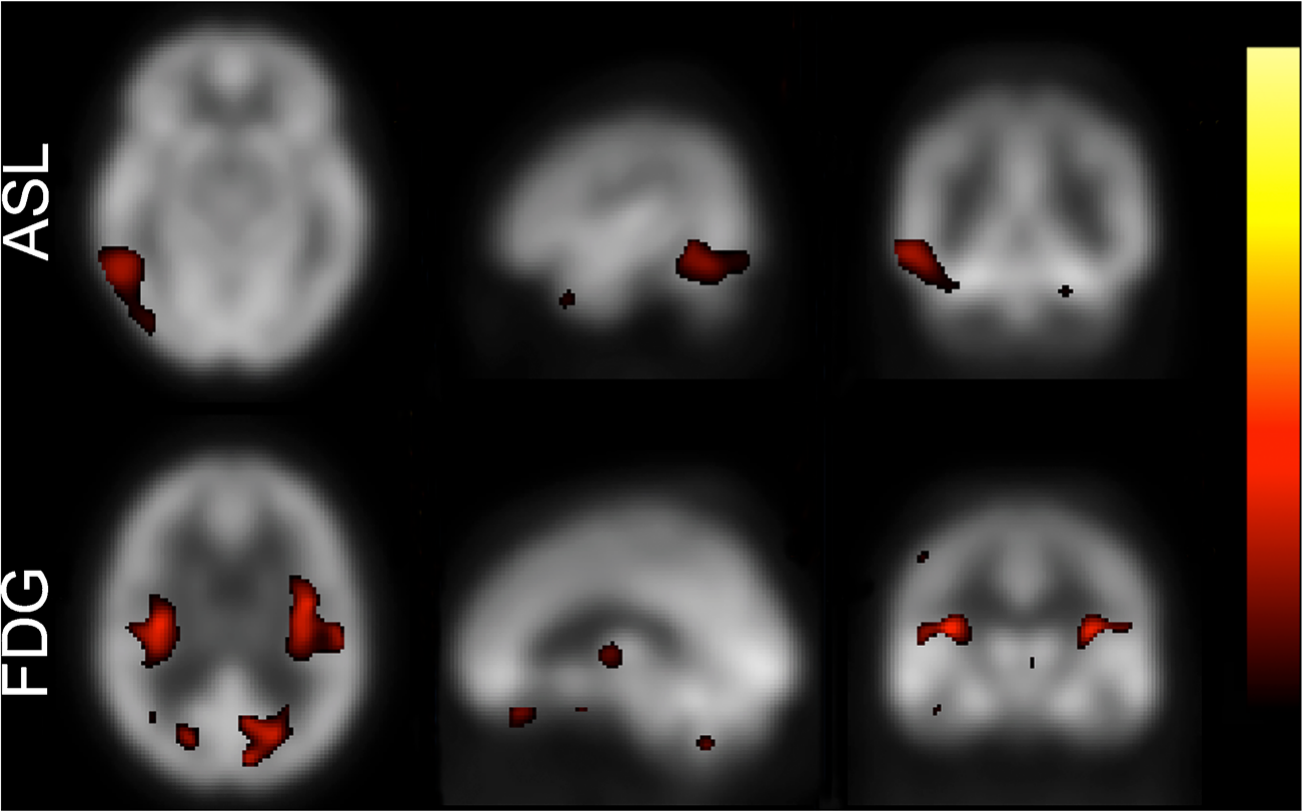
Healthy control with false-positive findings. Both the ASL-based and FDG-PET-based Z-score maps show false-positive Z-score patterns representative for the respective modality in this study. The clusters at the junction between grey and white matter in the lower row were present in several of the FDG-PET images of healthy controls, contributing to the number of uncertain cases, false-positive assessments and limited inter-rater agreement. The ASL-based map from this particular healthy control was read as AD by one reader, and the FDG-PET-based images as AD by two readers and FTD by one reader. The Z-maps are shown with thresholded overlays in a dichromatic colour scale with the default lower and upper Z-score thresholds of 2.0 (red) and 6.0 (yellow). *ASL* arterial spin labeling, *FTD* frontotemporal dementia, *PET* positron emission tomography, *AD* Alzheimer’s disease
